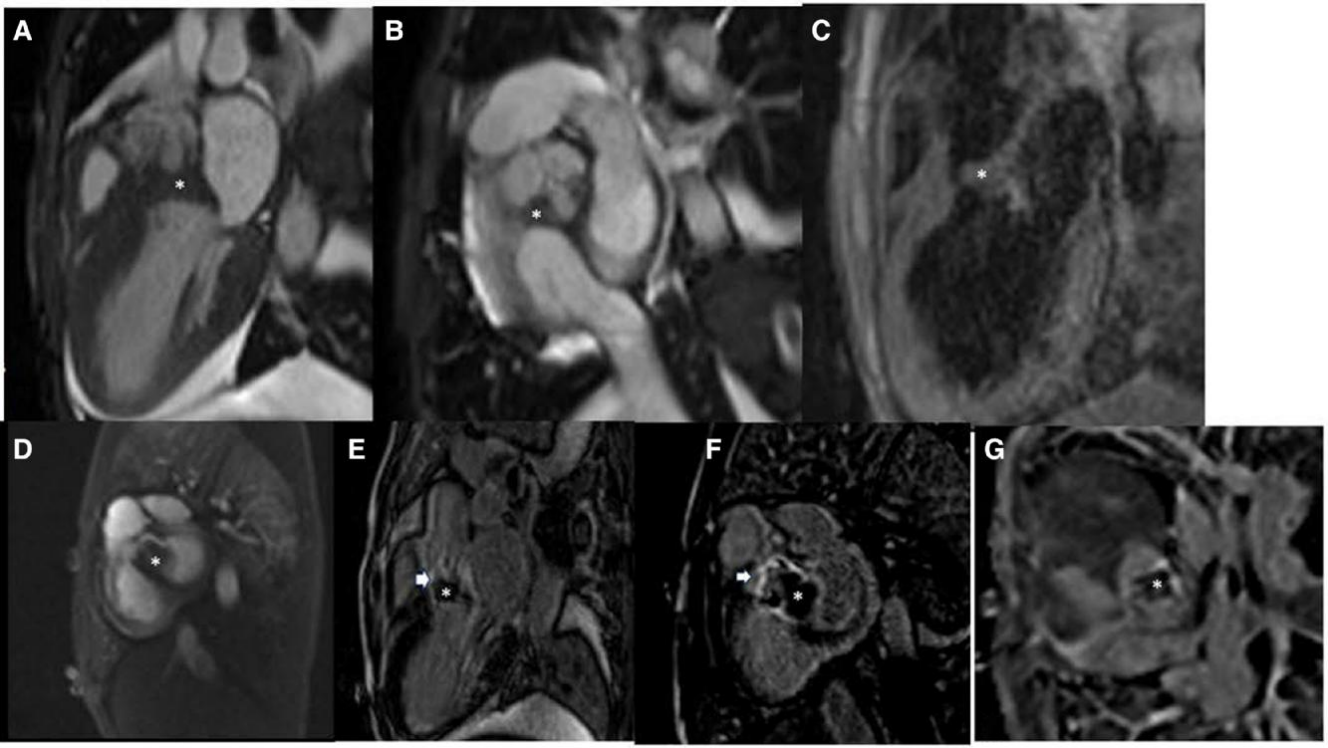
Cardiac MRI. (*A*)–(*G*) showing left ventricular outflow tract mass (*). (*A*) (left ventricular outflow tract view) and (*B*) (short-axis view) showing mass in the left ventricular outflow tract with aortic valve involvement. (*C*) (T2 dark blood sequence) showing isointense nature of the mass with myocardium. (*D*) (first-pass perfusion) showing no enhancement of the mass. (*E*) and (*F*) showing peripheral enhancement of the mass (white arrows). (*G*) is high TI (800 ms) sequence showing hypointense central core of the mass, suggestive of thrombus.
